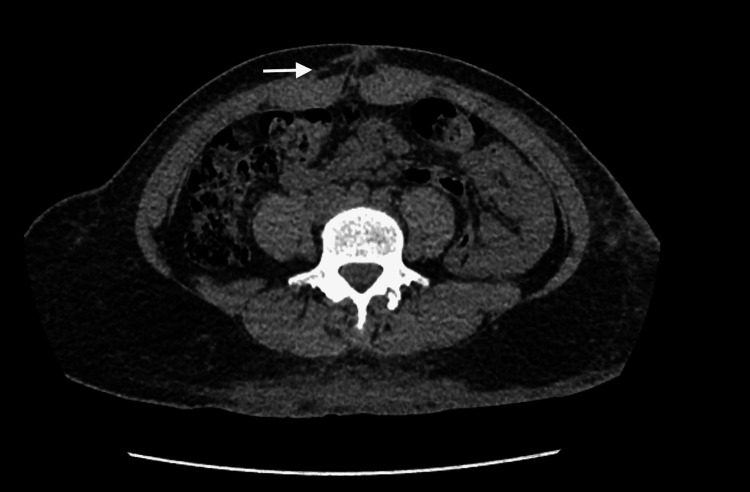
CT abdomen and pelvis The arrow shows abdominal wall subcutaneous stranding is unchanged no evidence for abdominal wall abscess.
